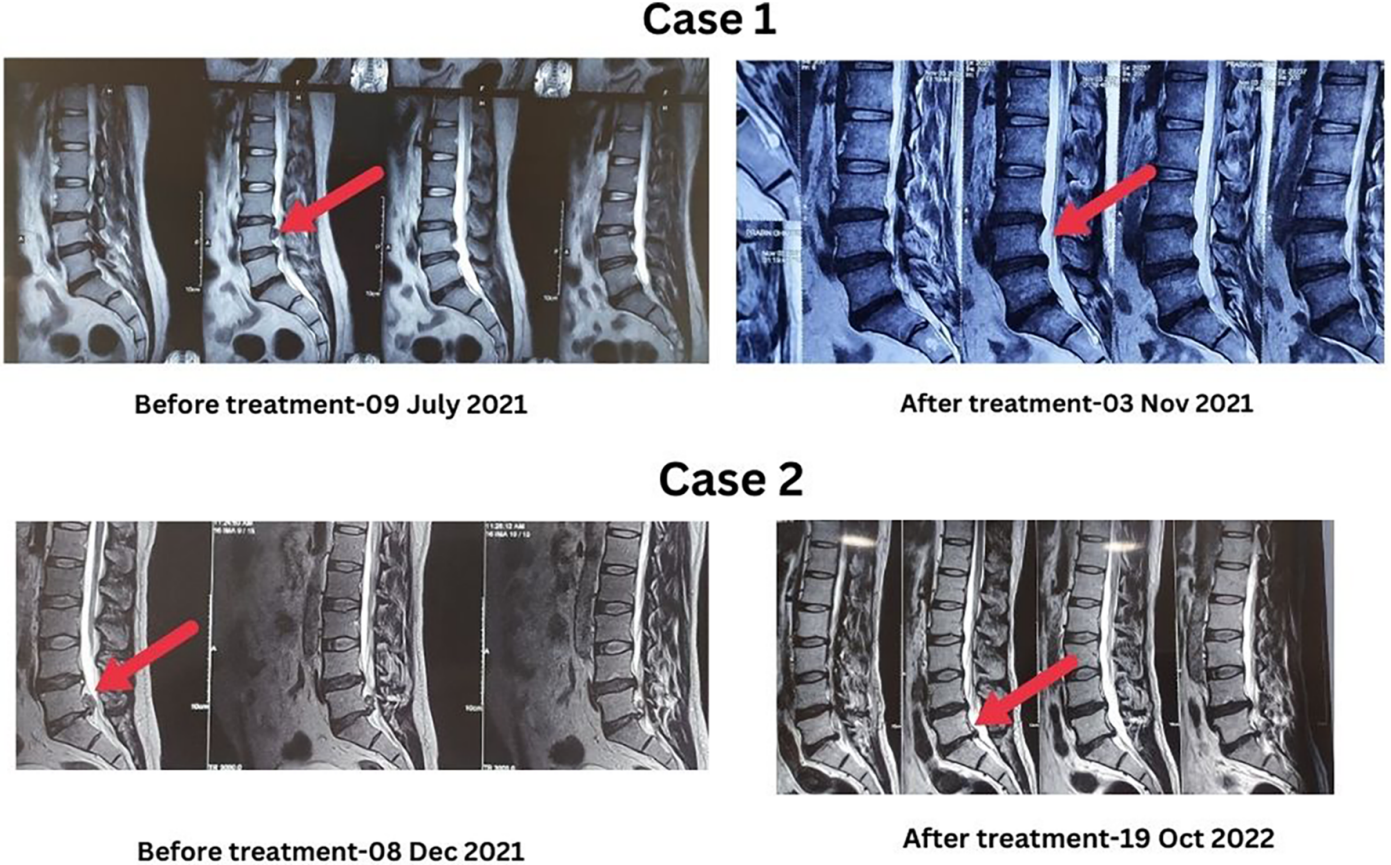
MRI changes before and after the treatments.
